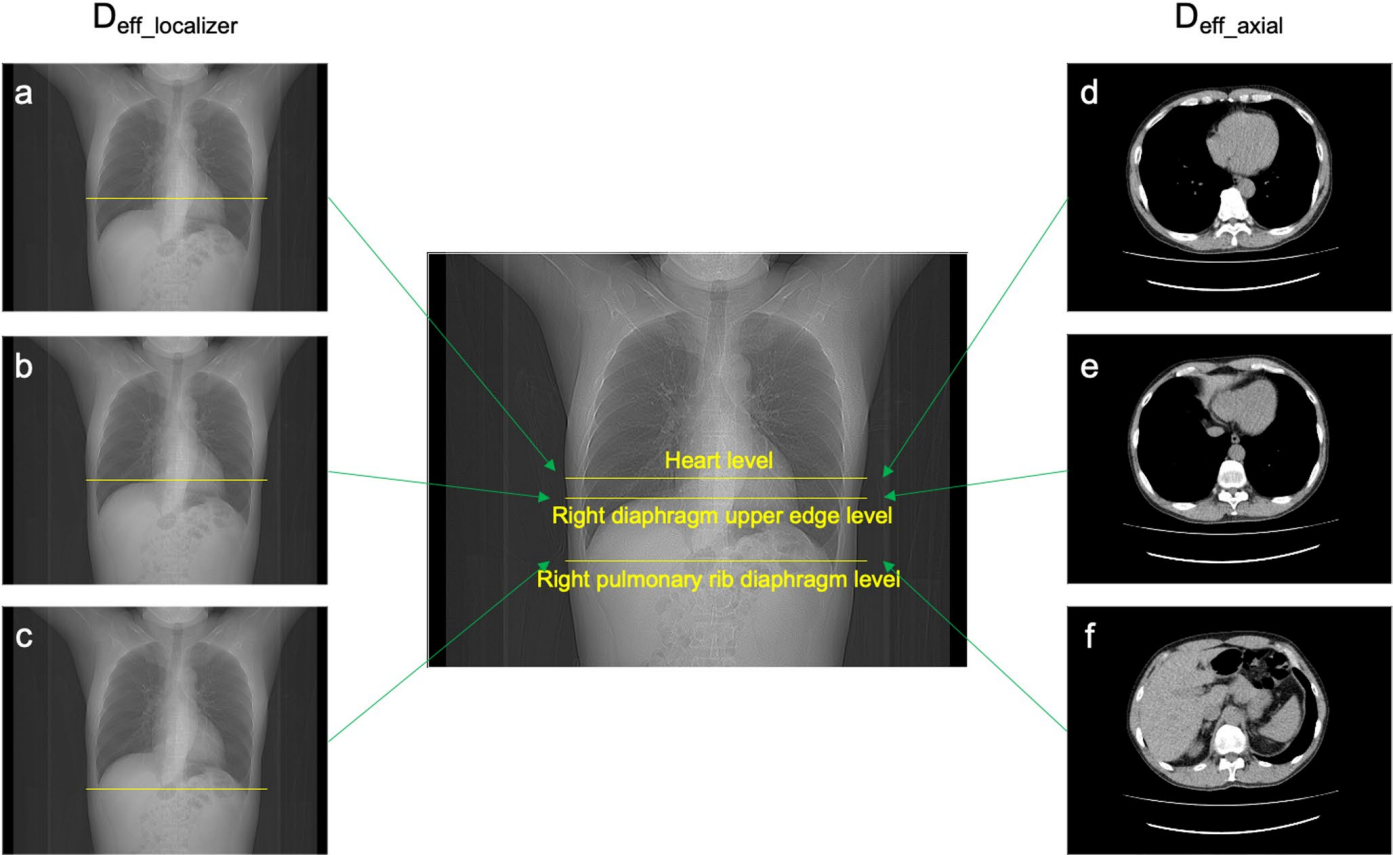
The D_eff_ at the heart, diaphragm upper edge, and right pulmonary rib diaphragm levels were measured in the localizer radiograph and axial images. The D_eff_localizer_ values measured from the localizer radiograph were transferred from the lateral diameter at the (a) heart, (b) right diaphragm upper edge, and (c) right pulmonary rib diaphragm levels. The D_eff_axial_ values measured from the axial images were transferred from the anterior-posterior and lateral diameters at the (d) heart, (e) right diaphragm upper edge, and (f) right pulmonary rib diaphragm levels. *D*_*eff*_ effective diameter, *D*_*eff_localizer*_ effective diameter value measured from the localizer radiograph, *D*_*eff_axial*_ effective diameter value measured from the axial image
